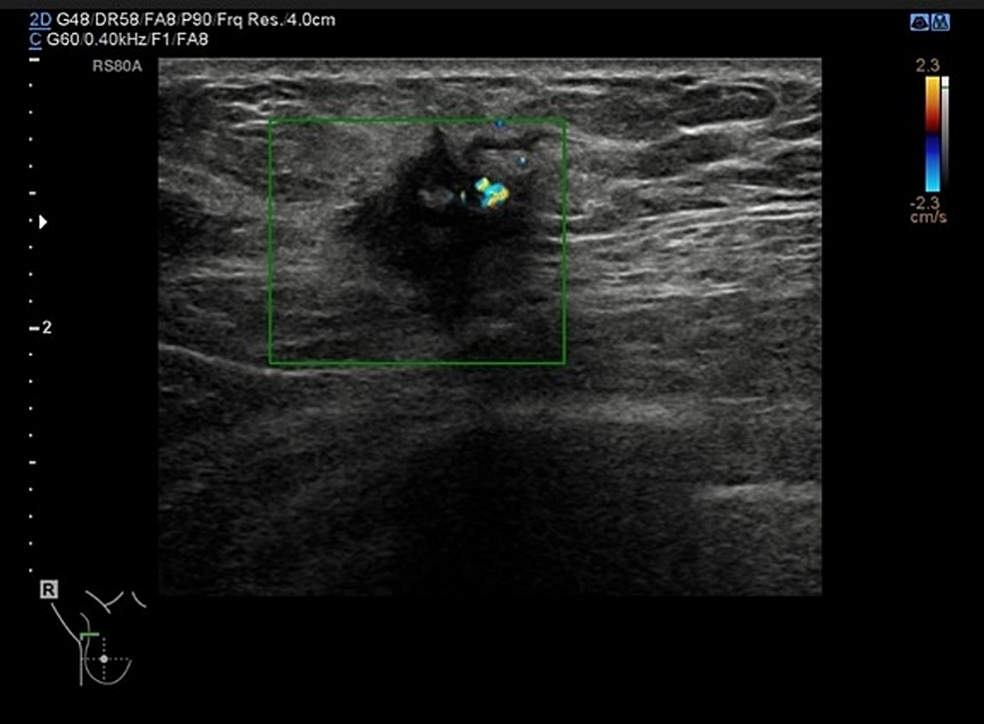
Right breast ultrasound showing a 25-mm suspicious lesion in the right breast axillary tail (green square).
